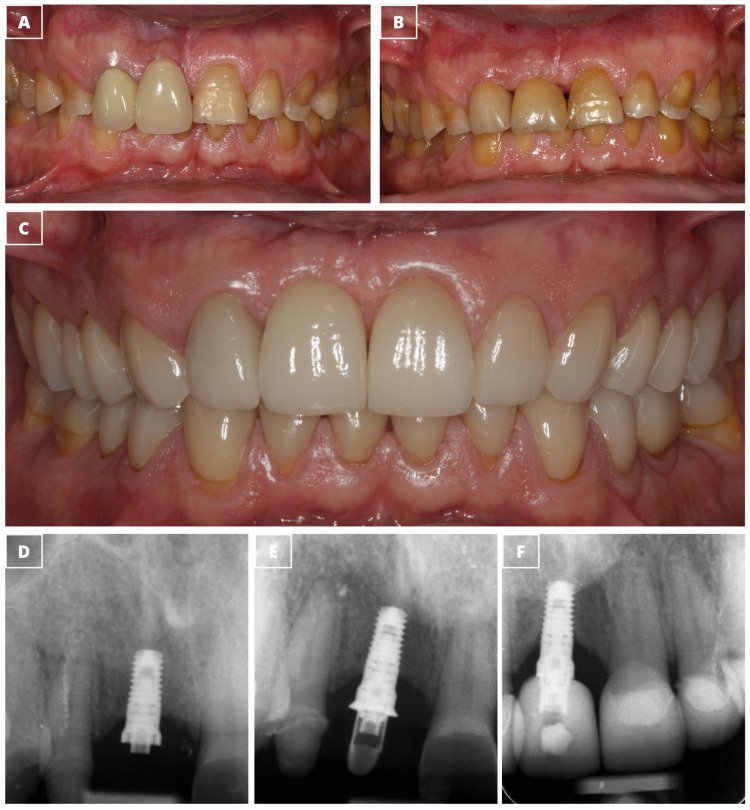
Clinical case treated by a single-unit narrow-diameter implant. (A) Clinical picture showing the baseline condition with missing #1.1 replaced by a fixed prosthesis. (B) The provisional prosthesis. (C) The definitive prosthesis. (D) Periapical radiograph showing the insertion of a 3.3 mm × 7.5 mm dental implant. (E) Periapical radiograph at the implant loading. (F) Periapical radiograph after four years of implant insertion.
